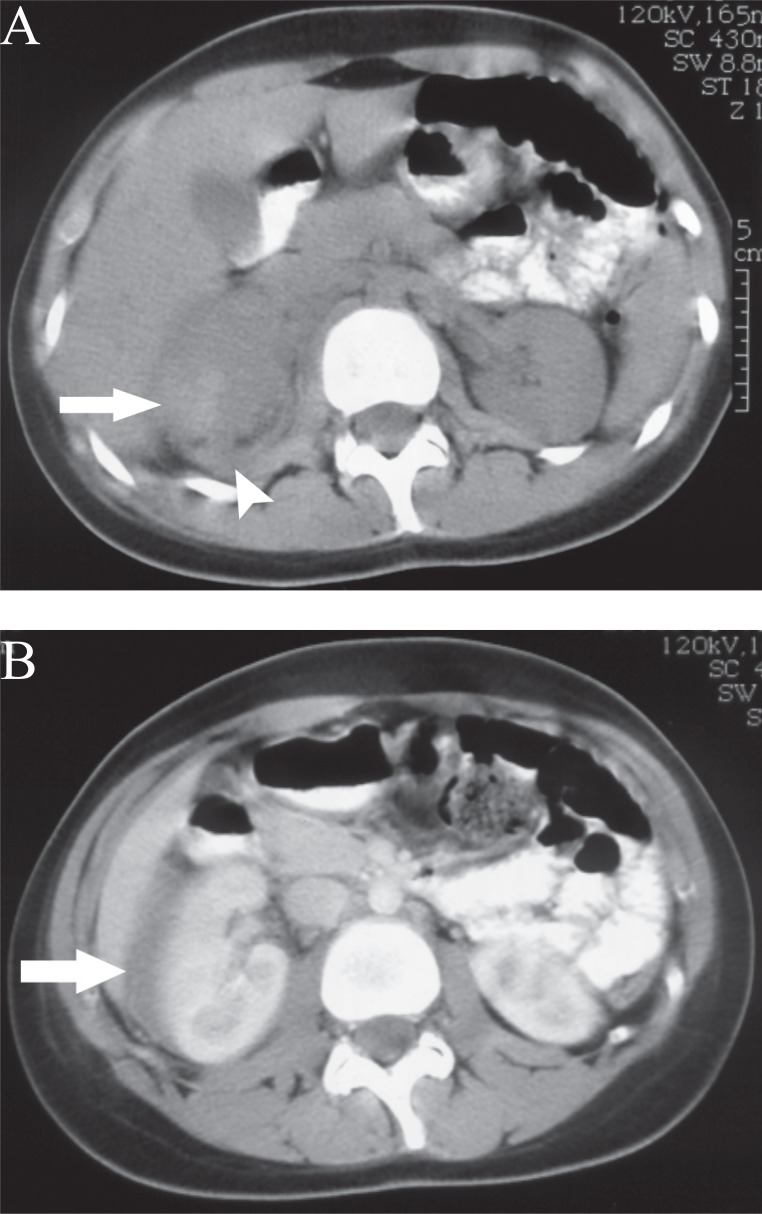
CT of right adrenal carcinoma. (A) On non-enhanced CT the hyperdense focus in the tumor (arrow) is seen. Extraadrenal haemorrhage (arrow head) is almost isodense with the tumor and with edema (arrow) surrounding the kidney seen on (B), that is contrast-enhanced CT.
